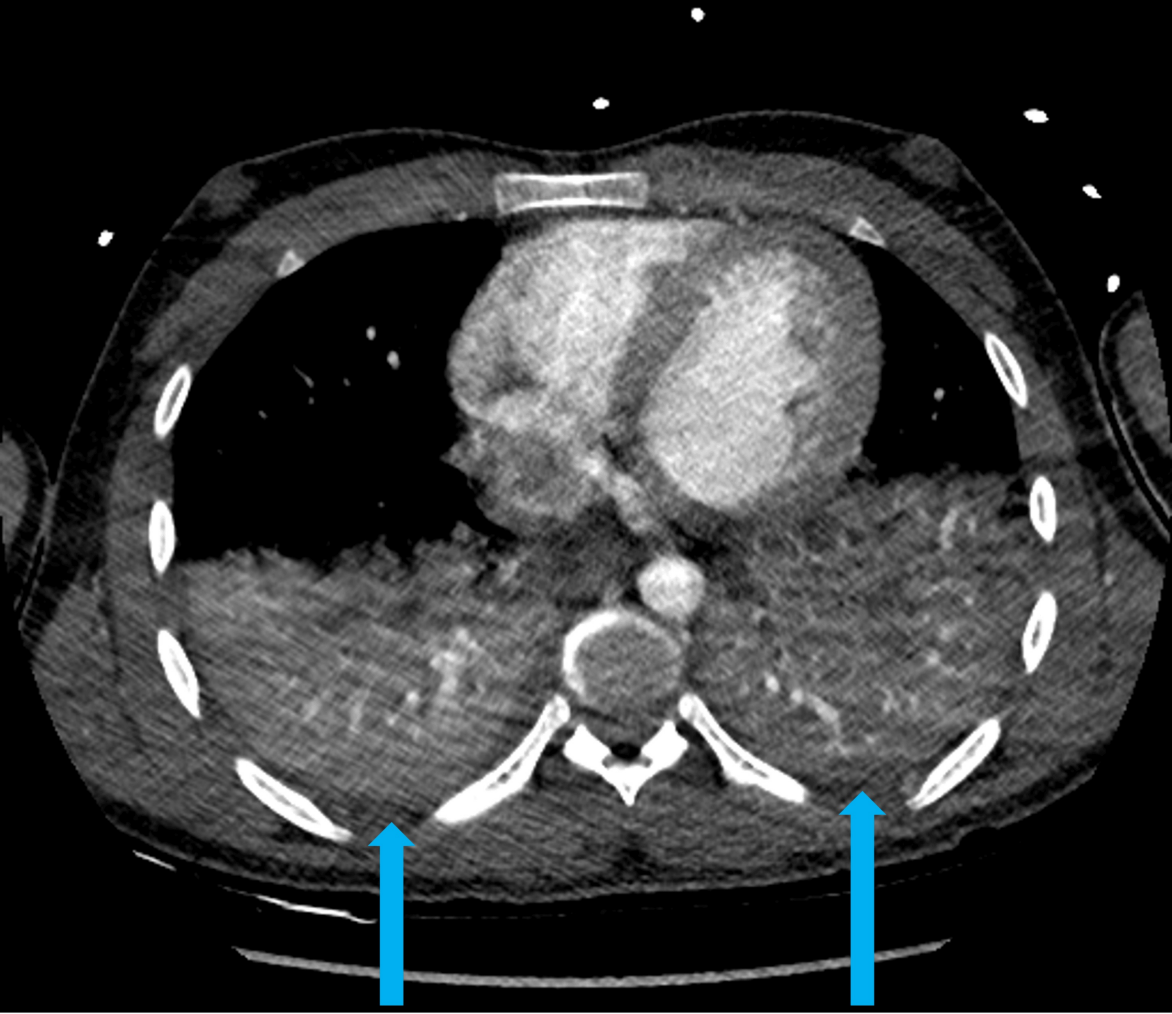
Chest computed tomography from hospitalization day 4 Blue arrows indicate large bilateral lower lobe consolidations.
